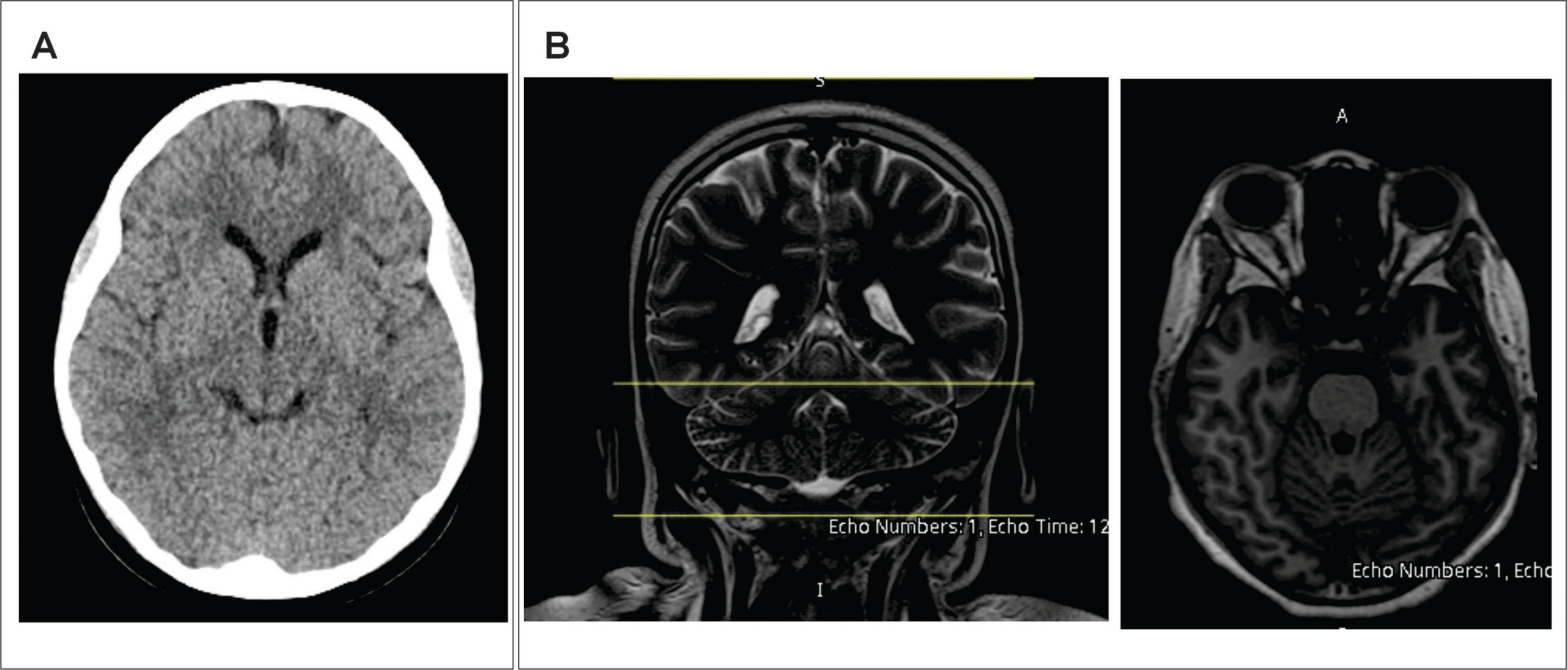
Neuroimaging during hypertensive encephalopathy. Non-contrast axial CT brain (A) and T2-weighted fluid-attenuated inversion recovery (FLAIR) MRI (B) performed during the acute neurological event. No evidence of hemorrhage, infarction, or vasogenic edema is seen. The findings were interpreted as normal.
